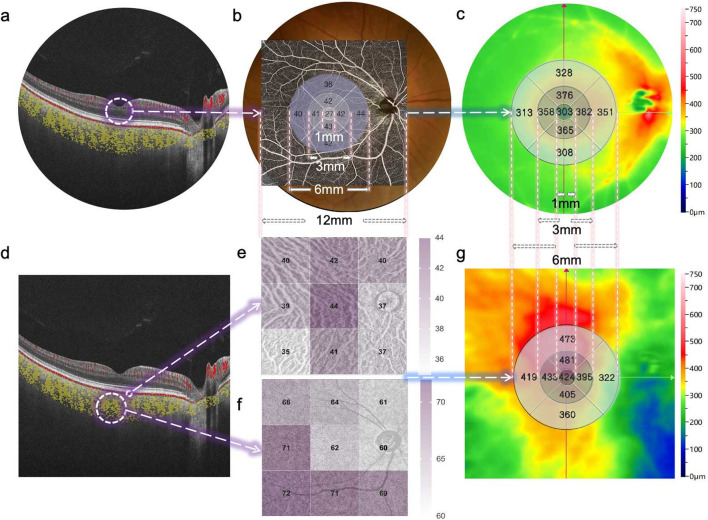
Representative SS-OCTA images obtained from a diabetic patient’s eye (12 × 12 mm area). (a) B-scan image of retinal layers. (b) Fundus photography of the retina and its corresponding macula center (CM) (1 mm diameter) and fan-shaped areas of 1–3 mm, 3–6 mm, and 6–12 mm on OCTA, as well as the quantified topography of the RVD in a total area of 6 × 6 mm. (c) The quantified topography of RT in a total area of 6 × 6 mm. (d) B-scan image of choriocapillary and ChV layers. (e) The quantified topography of CPV in nine subfields (superotemporal, temporal, inferotemporal, superior, central, inferior, superonasal, nasal, inferonasal) of the en-face image. (f) The quantified topography of CVI in nine subfields of en-face image. (g) The quantified topography of CT in a total area of 6 × 6 mm.
